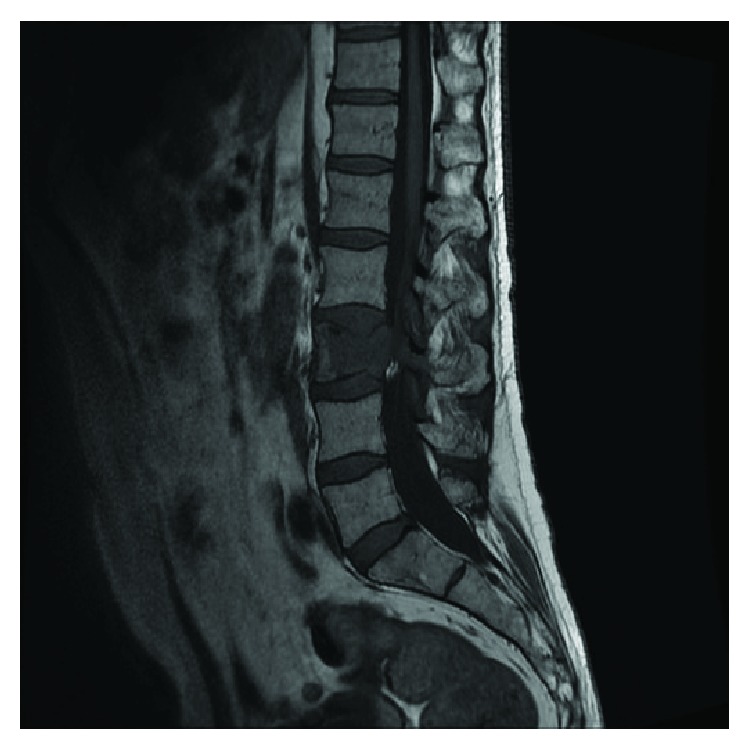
Sagittal T1-weighted MRI of the vertebral column without contrast enhancing showing tumor masses in the 3rd lumbar vertebrae (L3) with partial collapse of the corpus of L3 and tumor growth to the right paravertebral muscle.
